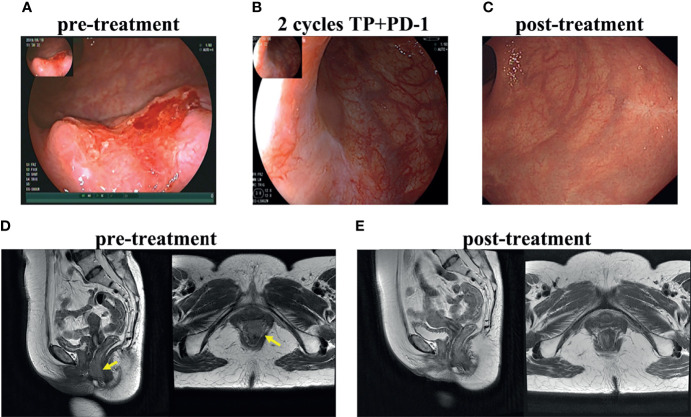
Treatment response of patient 1. Colonoscopy of primary tumor before treatment (A), after 2 cycles of neoadjuvant toripalimab combined with docetaxol and cisplatin (B), after radiotherapy and concurrent toripalimab (C). T2WI MRI image before treatment (D) and after the whole treatment (E).
